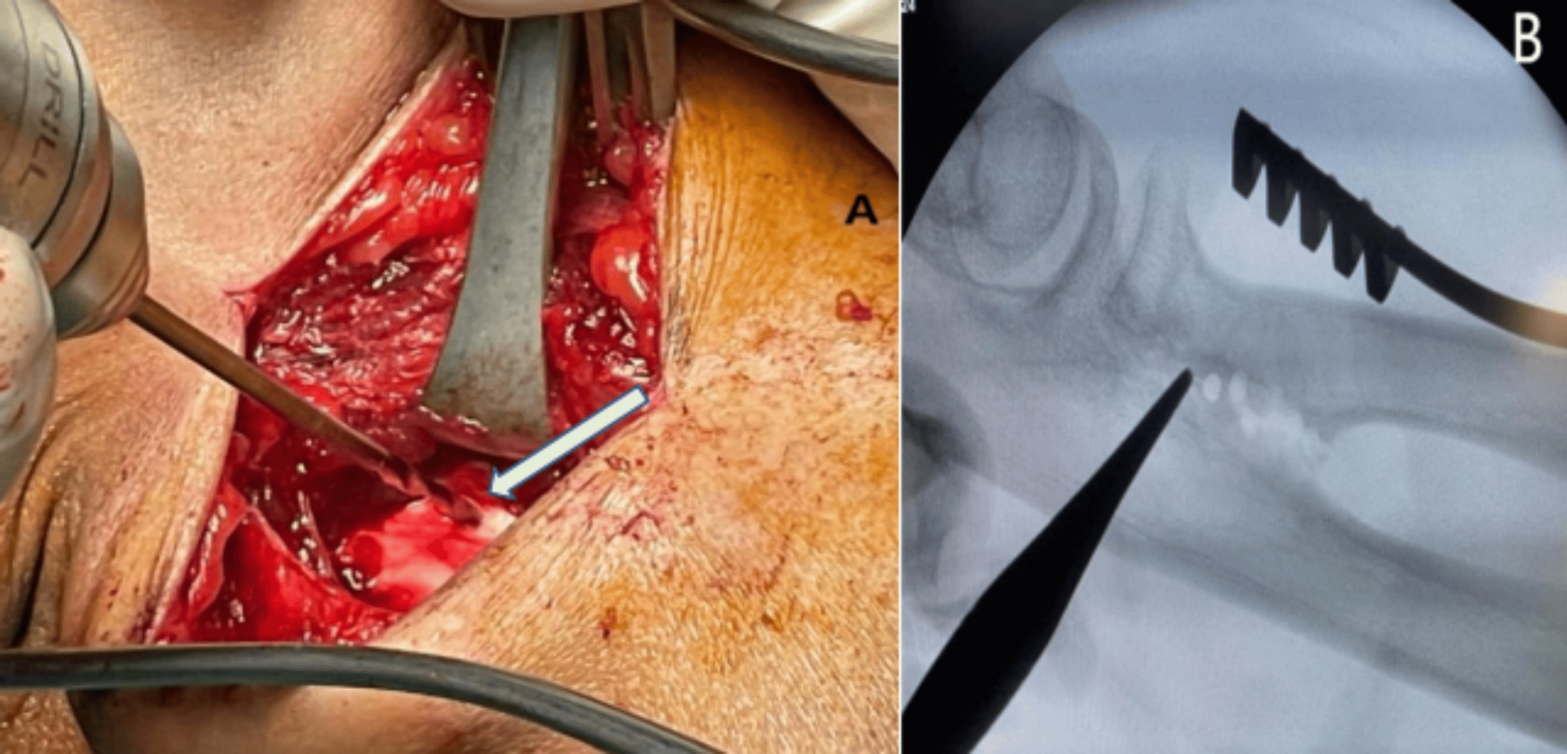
Intraoperative image, with the arrow showing the level of synostosis which was identified to be about 2 cm (A). Intraoperative radiography showing the level of synostosis which was located at the PRUJ level (B) PRUJ: proximal radioulnar joint
